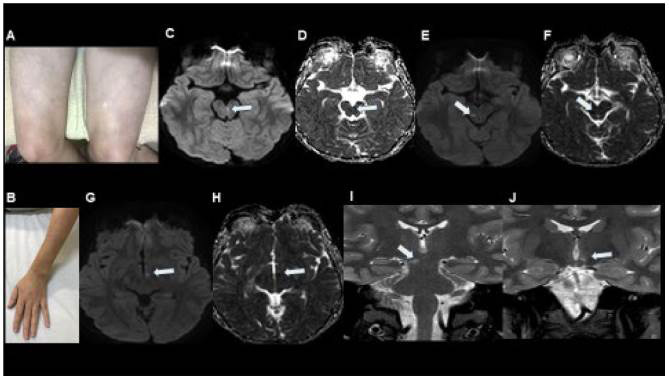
ADA-2 deficiency in 13-year-old boy with livedo reticularis (A, B) and sudden onset of left ophthalmoplegia. DWI and ADC mapping (C, D) shows restricted diffusion in the left paramedian portion of the mesencephalon in the projection of the left III cranial nerve fibers (arrows), compatible with acute stroke. One year later, this boy presented with another acute symptom, this time consisting of paresthesia and loss of taste in the left portion of the tongue. DWI and ADC mapping (E, F, G, H) showed restricted diffusion in the right paramedian portion of the mesencephalon and in the left hypothalamus (arrows), both compatible with acute stroke. Coronal T2-weighted images (I, J) confirmed these findings (arrows). Whole-exome sequencing was performed and showed heterozygosity for missense mutations in CECR1, thus encoding ADA-2.
